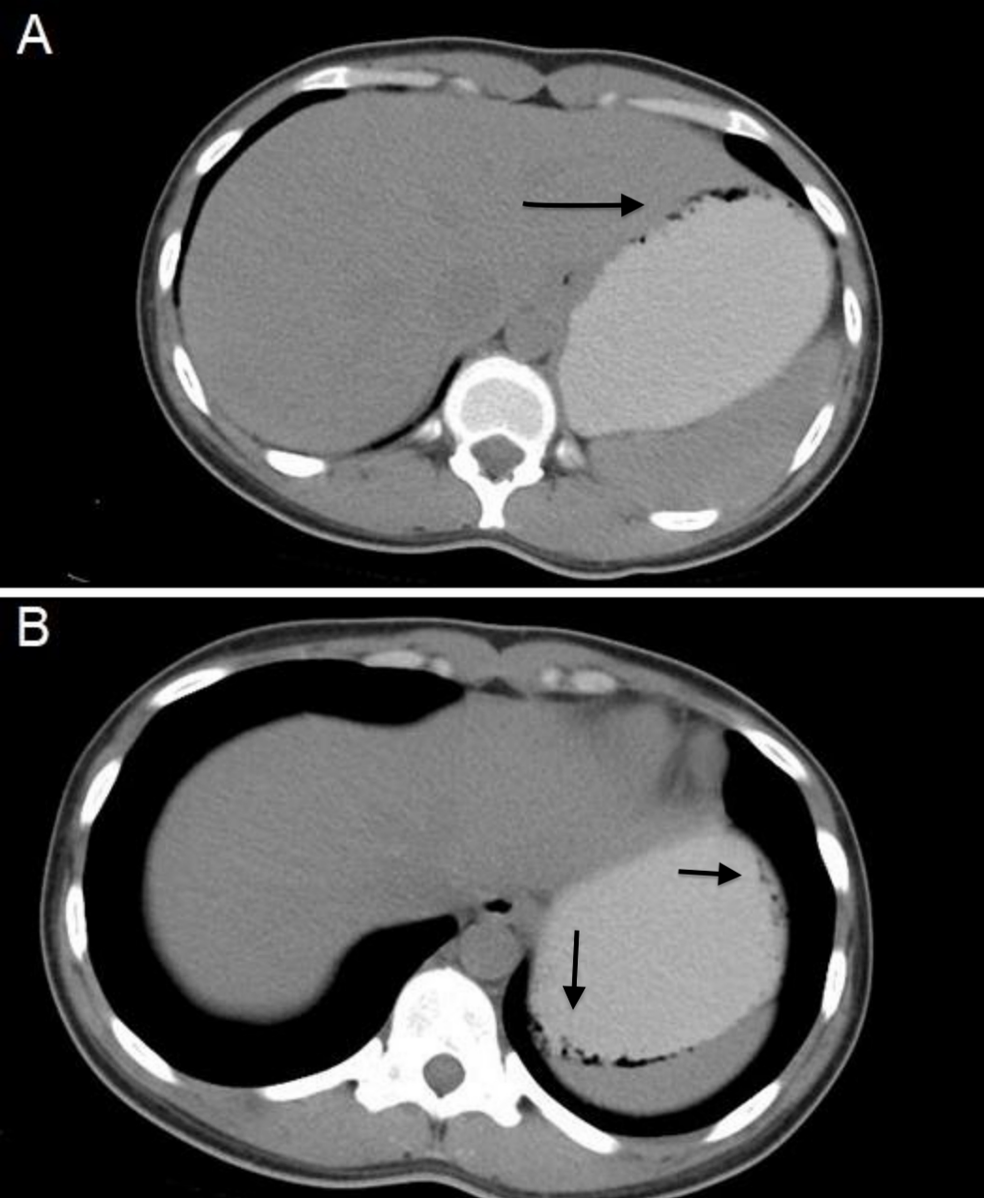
CT of the abdomen and pelvis with oral contrast A: CT of the abdomen and pelvis with oral contrast revealed bubbly gas within the gastric fundus and along the greater curvature concerning for gastric pneumatosis. B: CT of the abdomen and pelvis with oral contrast reported submucosal gas in the nondependent portion with trace submucosal gas identified in the dependent portion of the greater gastric curvature.
